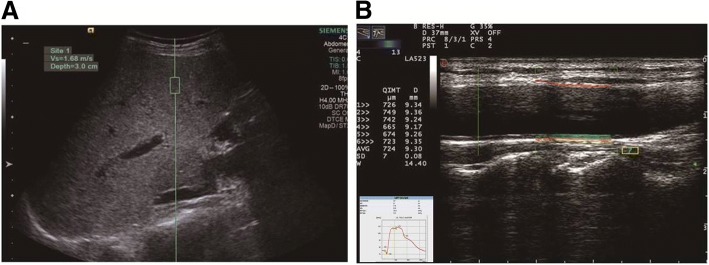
ARFI liver stiffness images and common carotid artery RF ultrasound images in patients with CHB: a Use of the ARFI technique to measure liver stiffness in patients with CHB using a conventional ultrasound interface. b Use of QIMT and QAS techniques to quantify the carotid artery intima-media thickness (IMT) and vascular elasticity in patients with CHB
